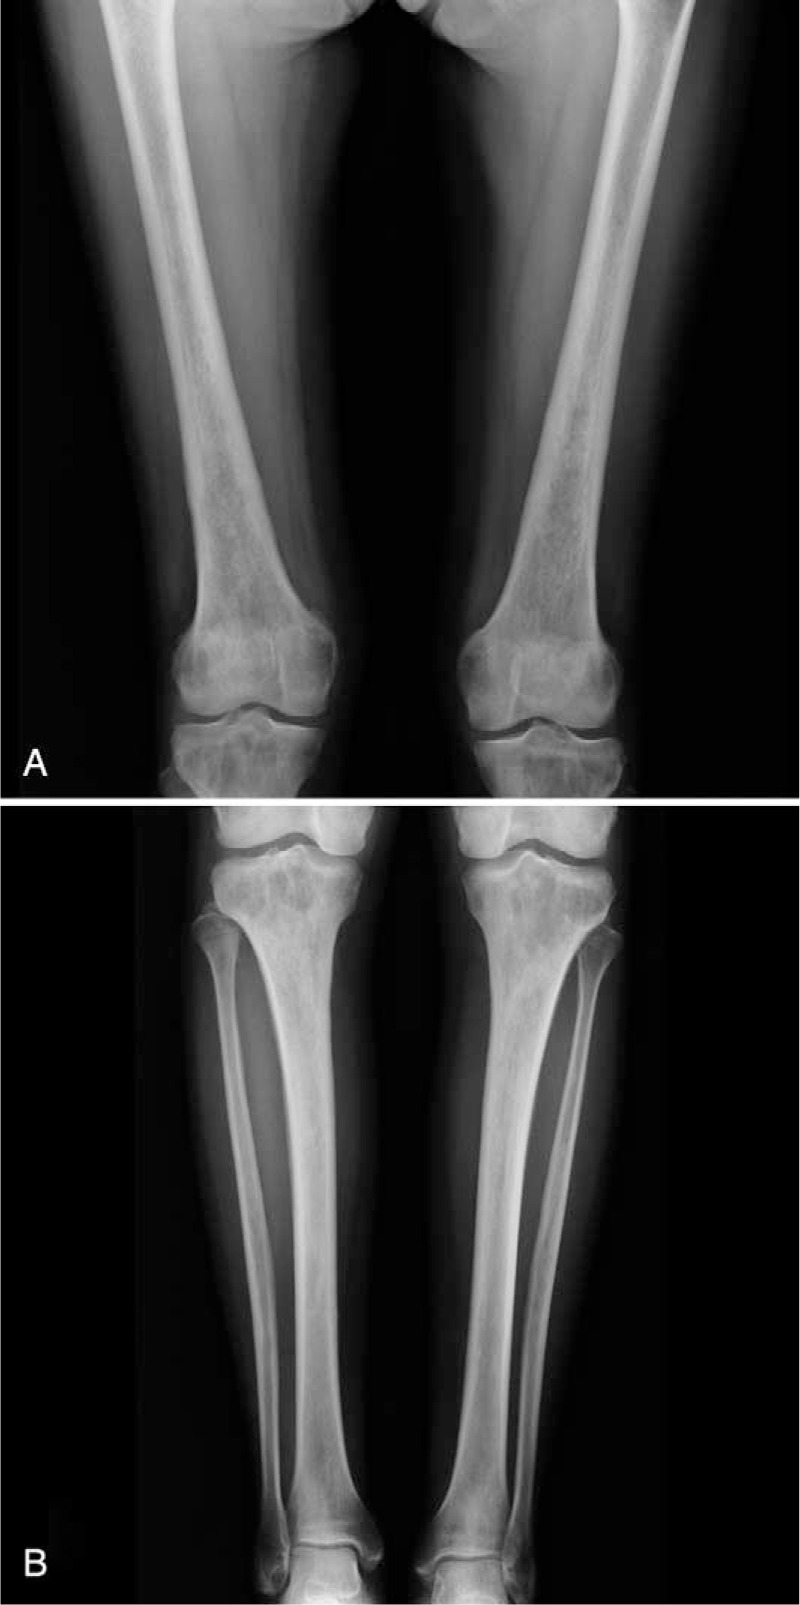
Femur (A), tibia and fibulas (B) radiography showing bilateral and symmetrical osteosclerosis of metaphysis and diaphysis.
